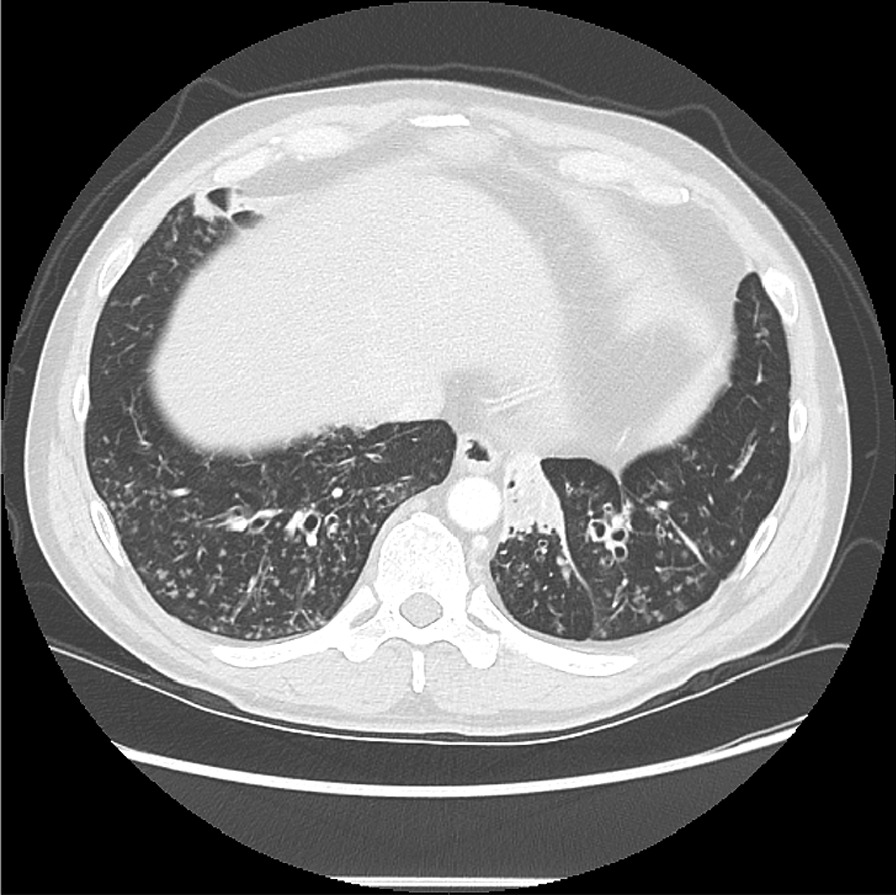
Representative CT cut of a subject with moderate bronchiectasis
